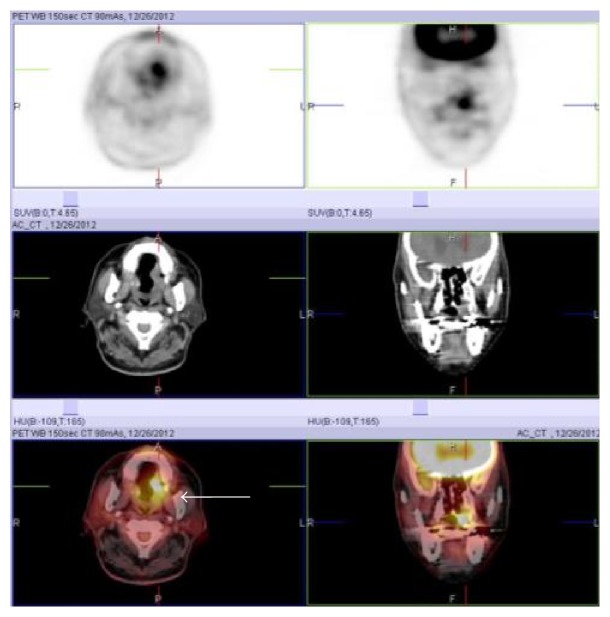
PET-CT image showing abnormal uptake of FDG (SUVmax = 5.2) in the left hard palate.
